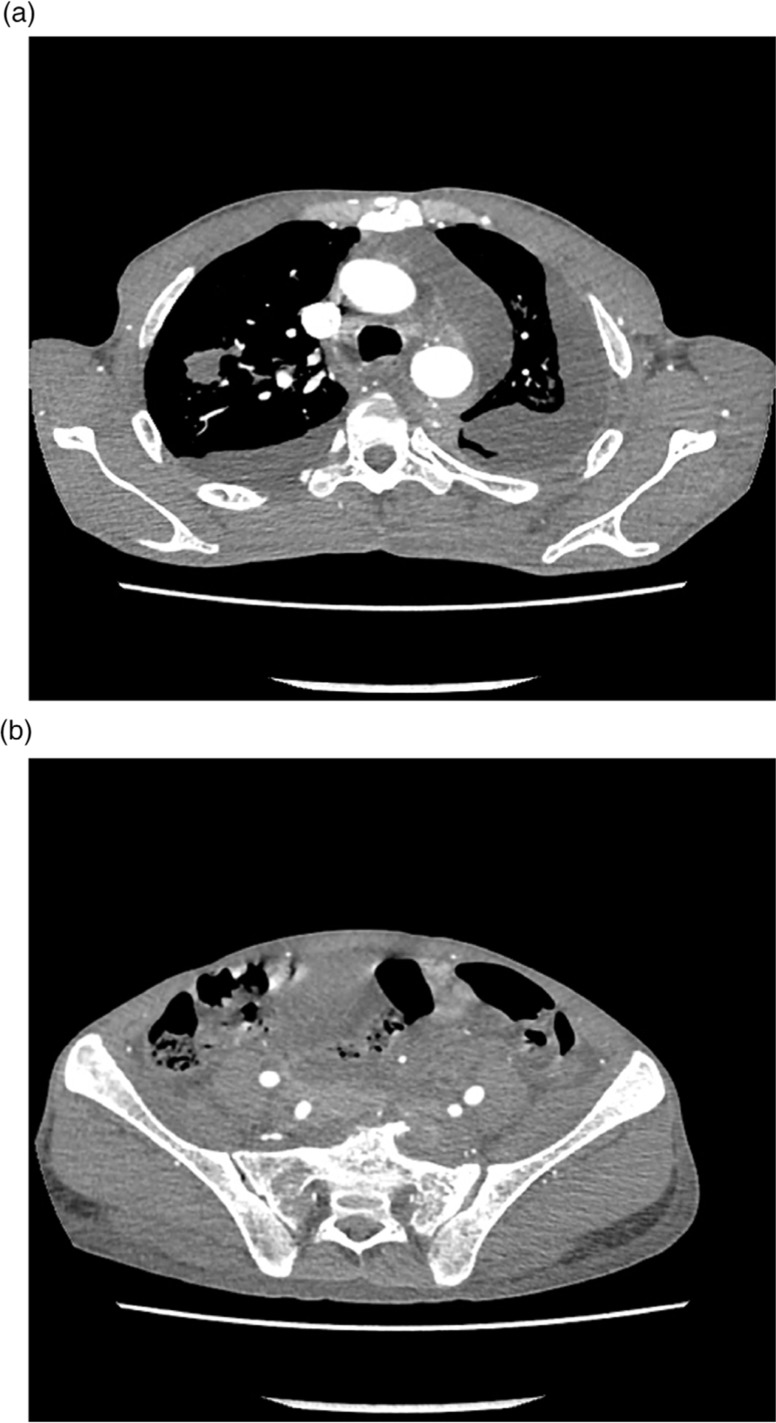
(a) Computed tomography scan of the chest with contrast showing bilateral effusion with pleural infiltration, right upper lobe involvement, soft tissue lesion in the posterior mediastinum. (b) Computed tomography scan of the abdomen showing lytic lesions in pelvis and sacrum.
